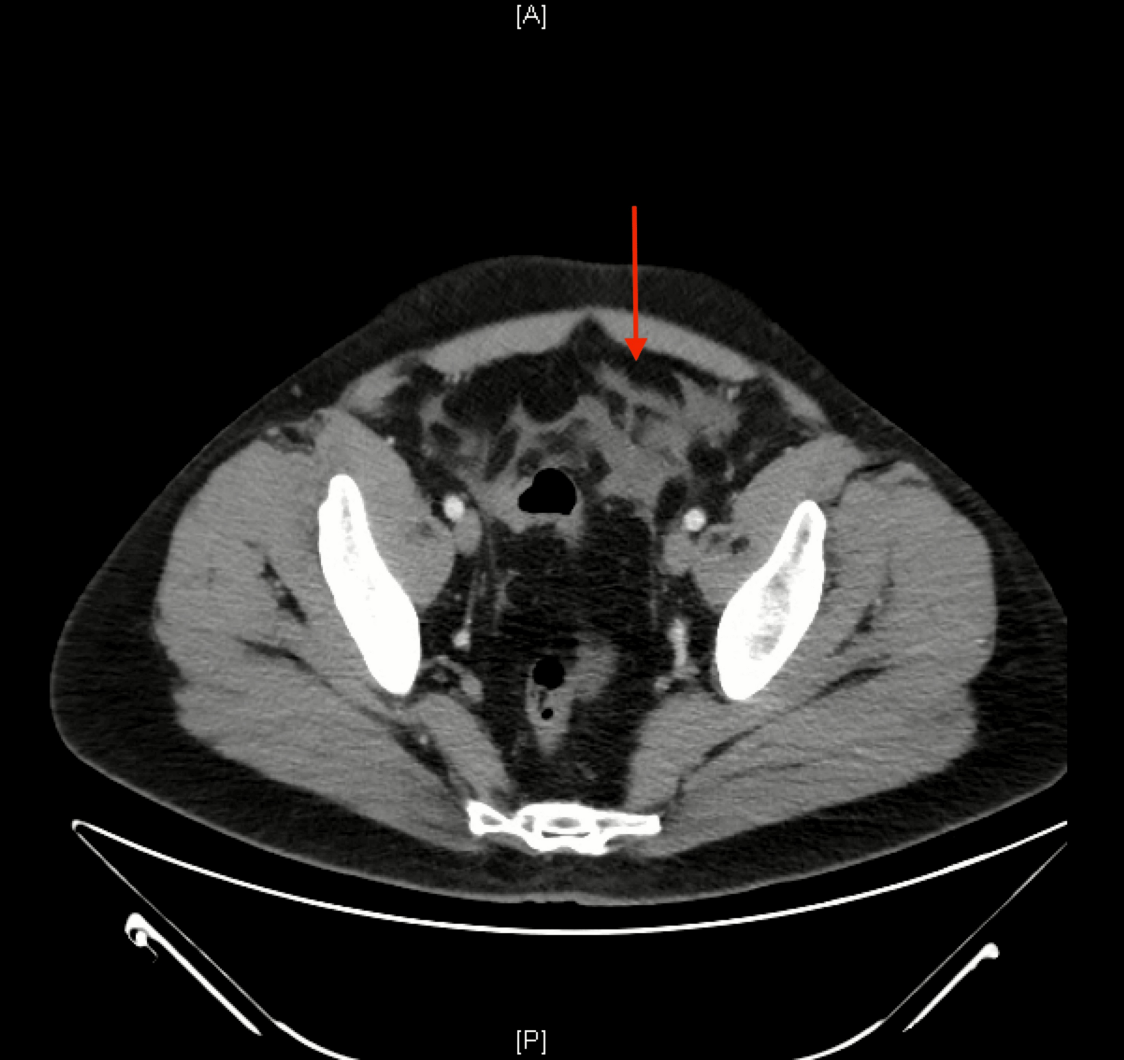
Axial CT scan of the pelvis The red arrow shows fat stranding and free fluid within the pelvic cavity. A: anterior; P: posterior
